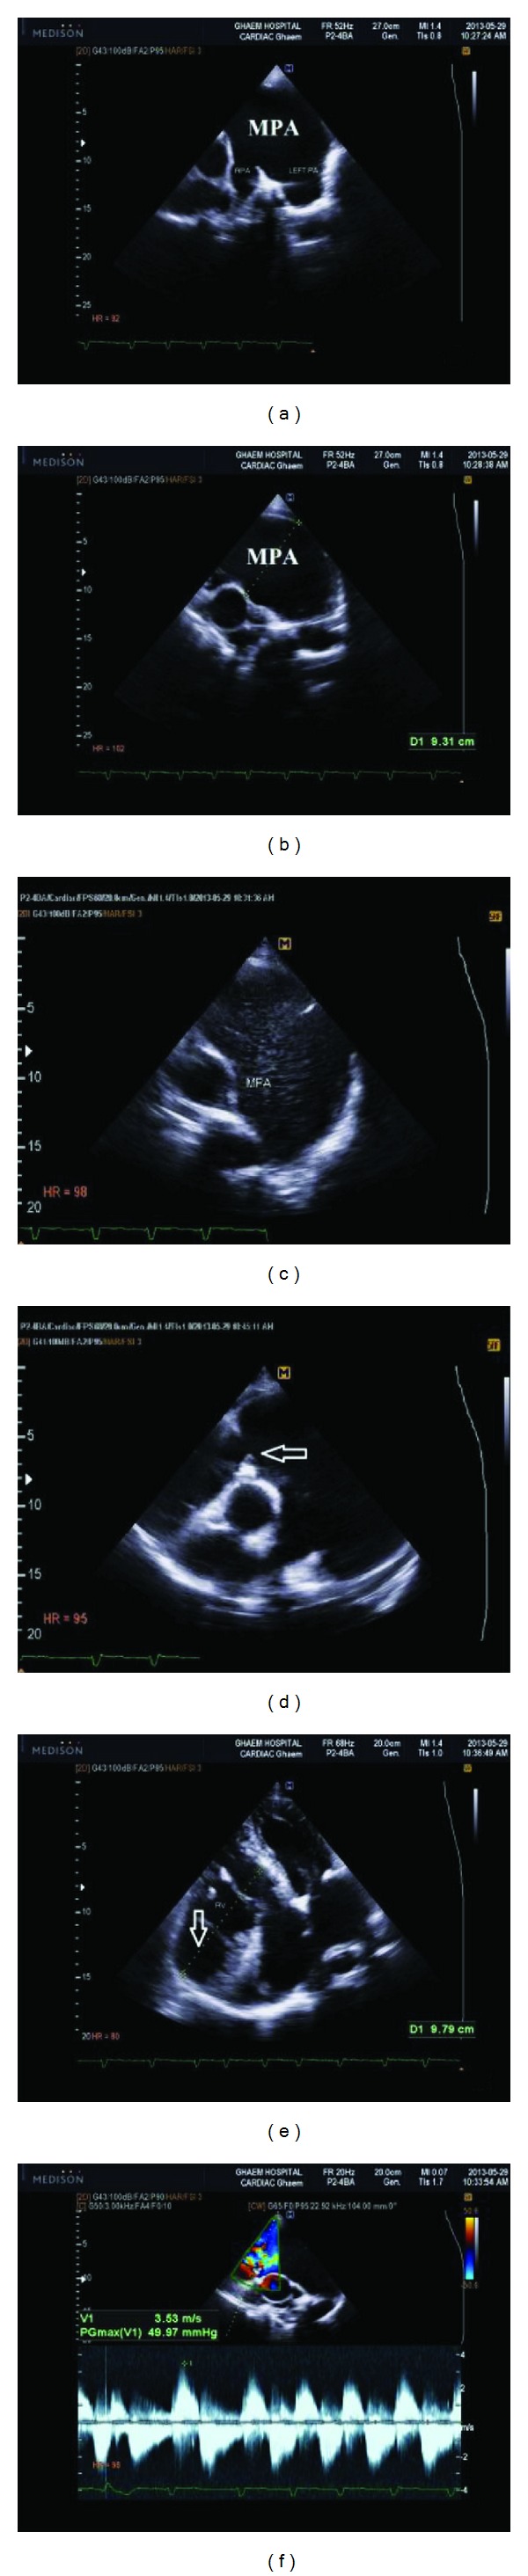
Patient's echocardiography: (a), (b), (c), and (d) parasternal short axis view, visible pulmonary valve (PV), annulus, and retracted leaflets with severe dilatation of main pulmonary artery and both branches (the arrow shows the dysplastic pulmonary leaflet); (e) apical 4 chamber view revealed severe aneurysmal dilatation of right ventricular inflow (the arrow); (f) doppler study of pulmonic valve from high left parasternal view. RPA: right pulmonary artery. PA: pulmonary artery. MPA: main pulmonary artery. RV: right ventricle.
